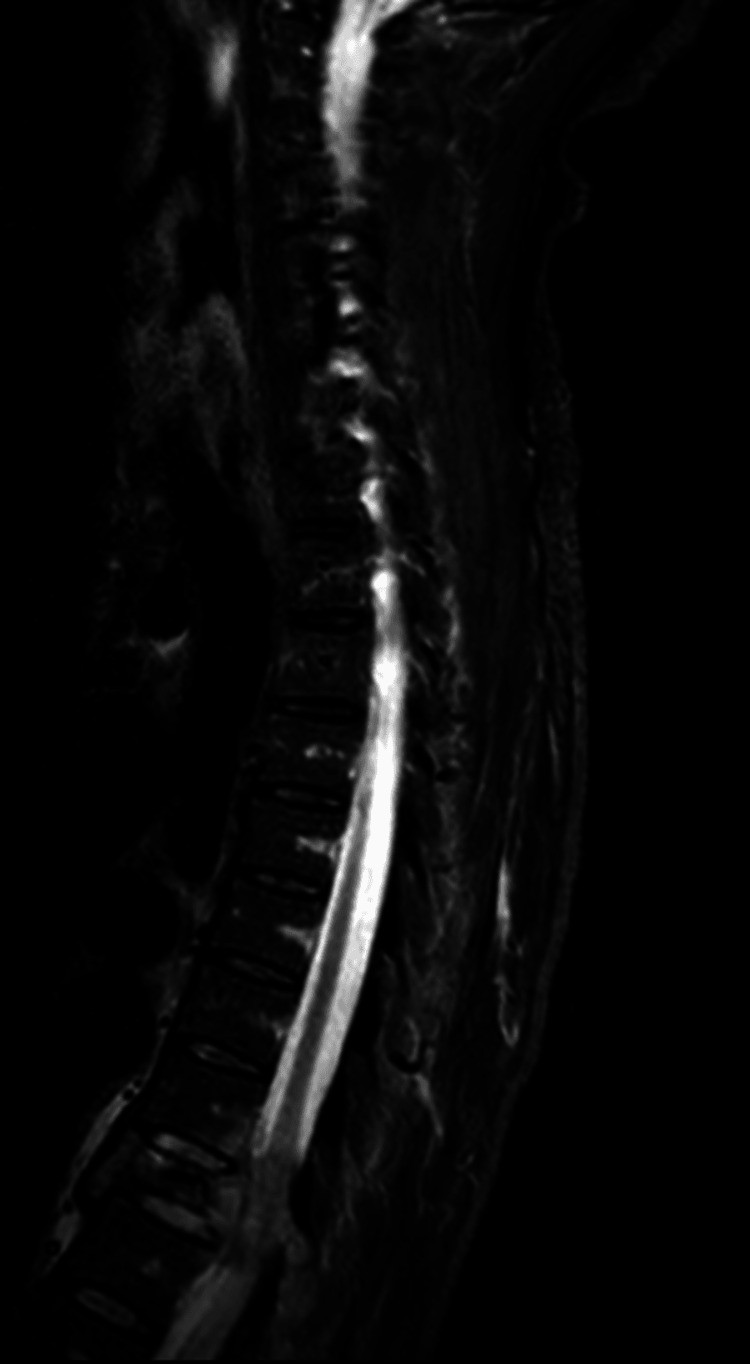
MRI of the thoracic spine showing a pathological fracture at T9 with an associated destructive soft tissue mass causing marked SCC SCC, spinal cord compression
